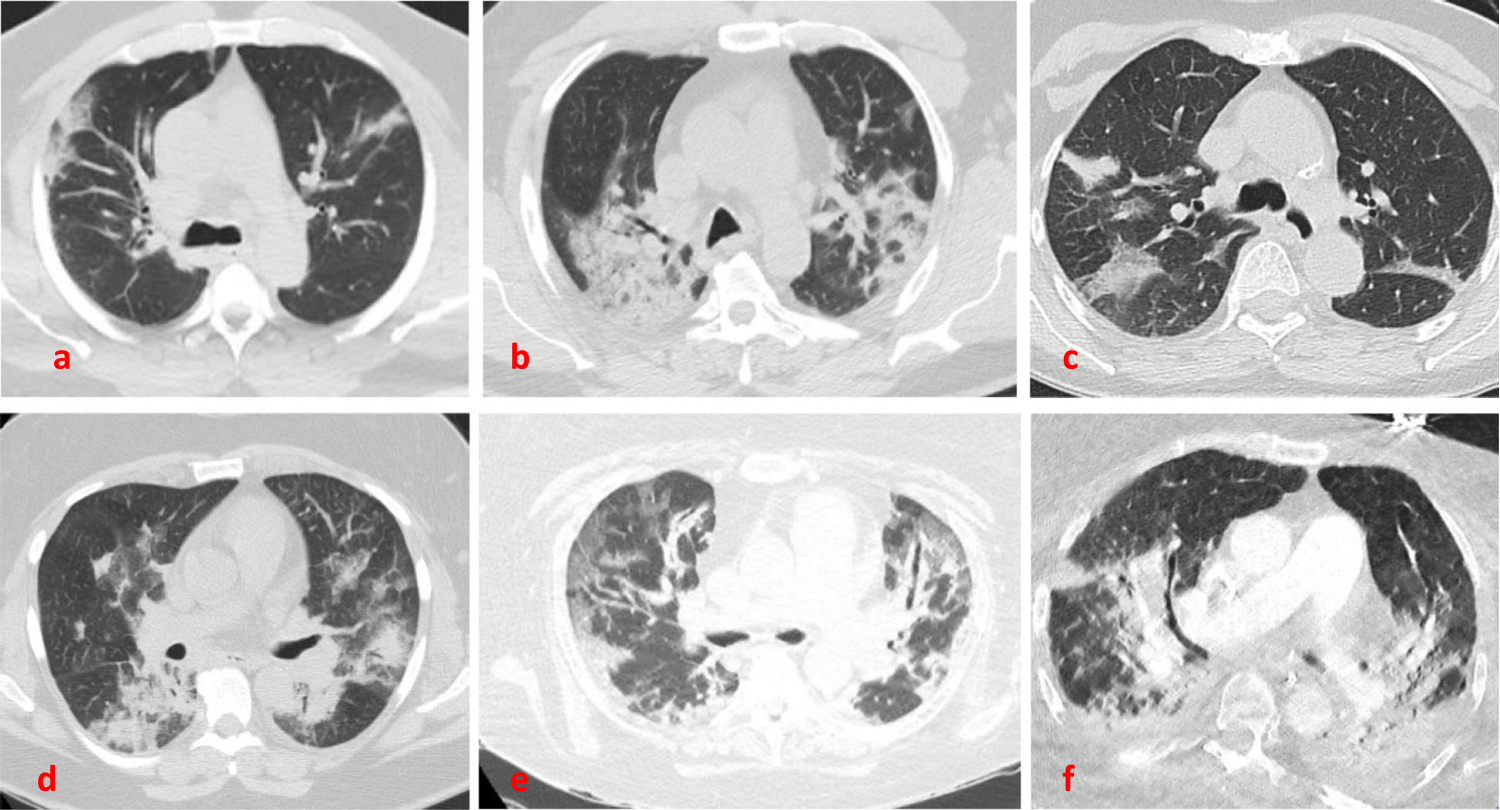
Baseline CT scan of the chest. Areas of GGOs, consolidations and crazy paving seen commonly bilaterally, more predominant in the posterior and peripheral lung regions. From top left: a Case-1. b Case −2. c Case −3. d Case-4. e Case-5. f Case 6
